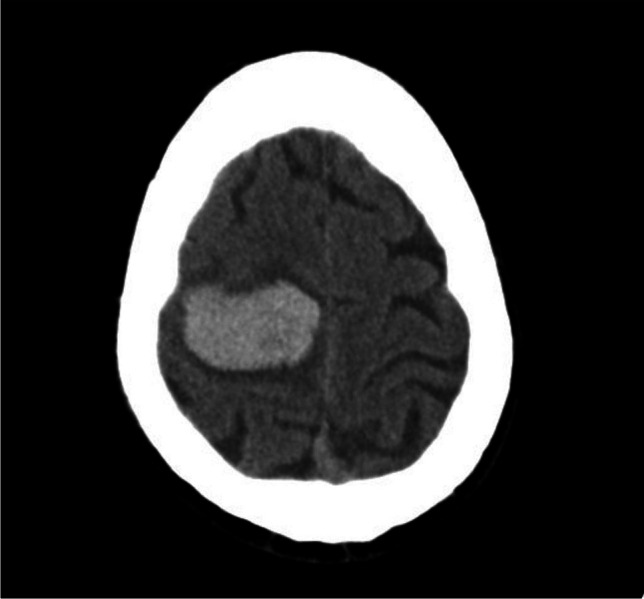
Axial non-contrast head CT demonstrating intraparenchymal hemorrhage in the posterosuperior aspect of the right frontal lobe
